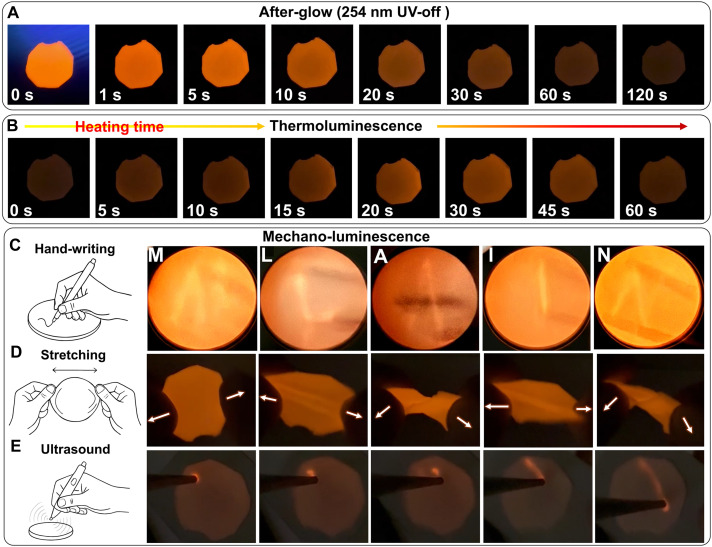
Visualization and application potential of the PL-, TL-, and ML-active AlN:Mn^2+^-modified PDMS film. (A) Afterglow emission, (B) TL, and (C to E) ML-based applications of the AlN:Mn^2+^-modified PDMS film. Bright orange ML effects in [(C) to (E)] were induced with different stimuli, i.e., friction (C), stretching (D), and ultrasounds (E), using a pen-like metal rod, hands, and FUS device, respectively.
